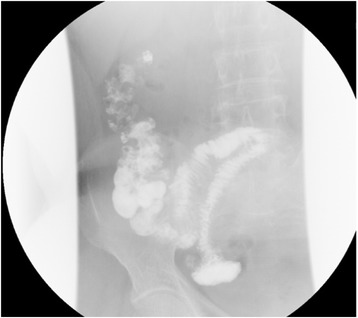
The digital radiography showed that the distal ileum approximately 40cm from the ileocaecal junction was entrapped
